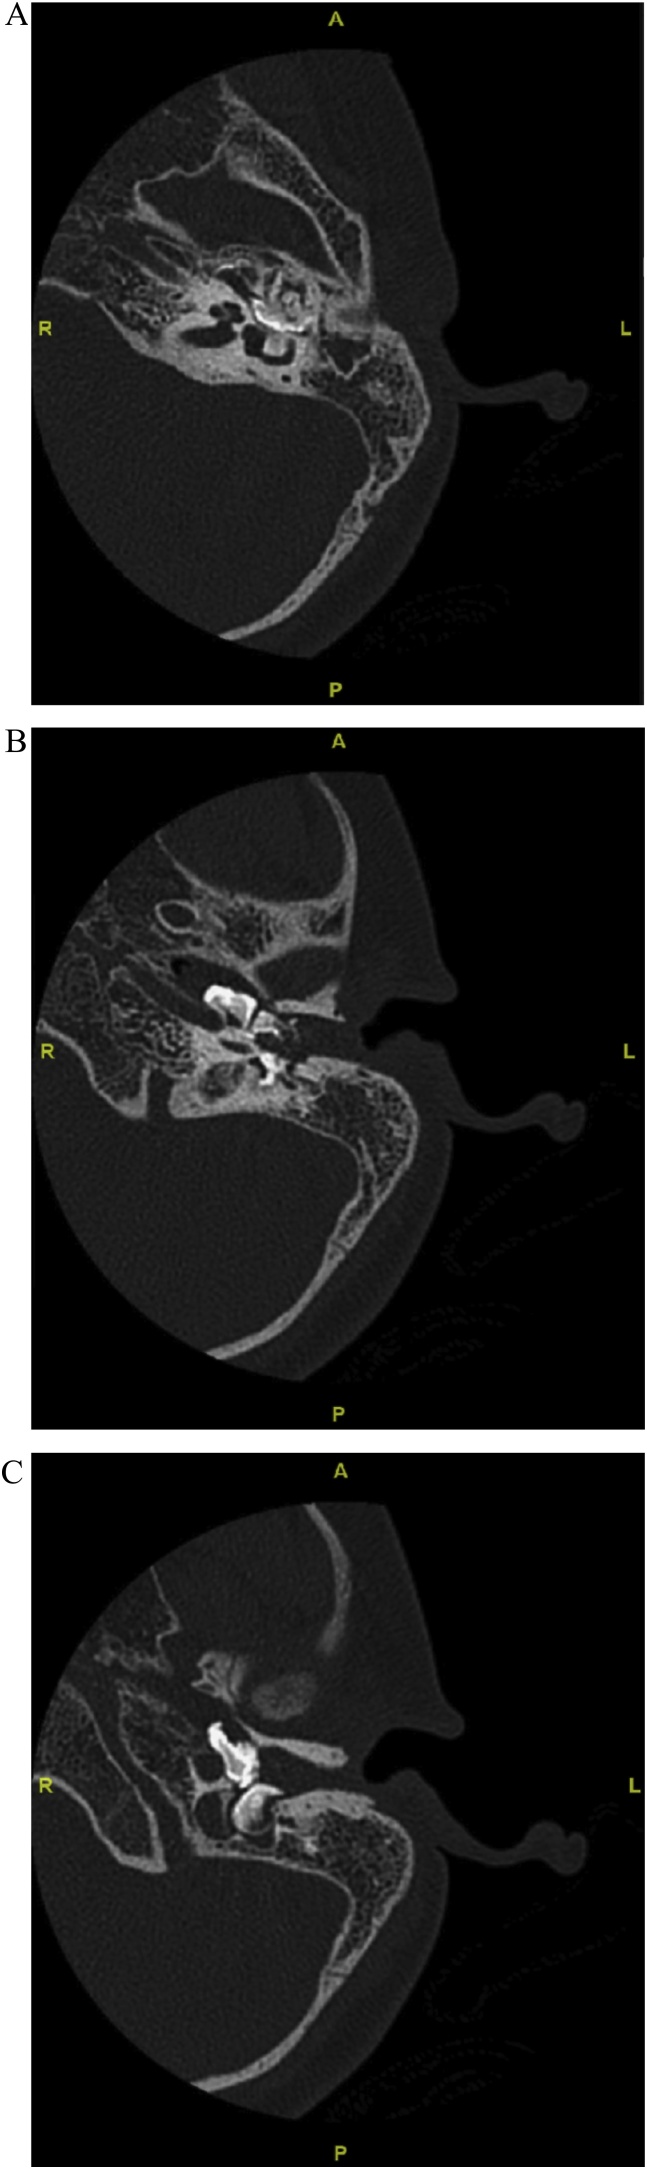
A. Computed tomography scan axial cut of the left mastoid area showing middle ear hyperdense mass compressing on tympanic segment of facial nerve and lateral semicircular canal. B. Lower down cut showing 2 odontomas, the anterior one in direct contact with carotid canal and eustachian tube. C. the lowest cut showing the same 2 odontomas one in contact with carotid artery while the posterior one compressing on the jaguar bulb.
